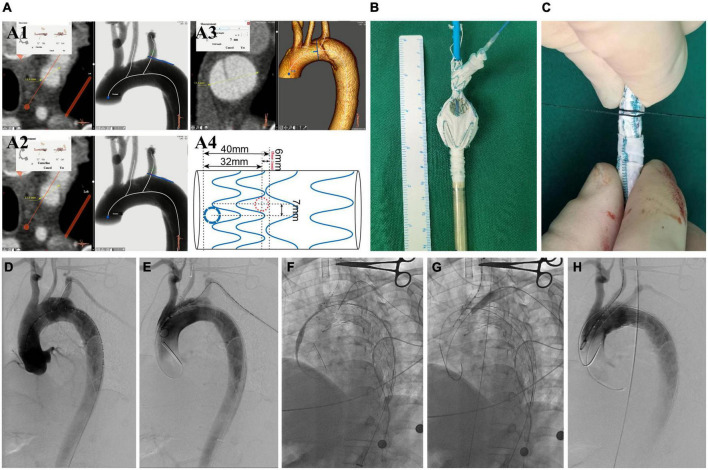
The surgeon’s details modified fenestrated stent graft and the intraoperative images of performing this endovascular technique. (A1) Measuring the diameter of LCCA and the offset angle between LCCA and LSA. (A2) The diameter of LSA. (A3) The distance between the tear entry and the proximal side of the stent. (A4) A design for preparing the modified fenestrated stent graft. (B) The unsheathed custom-made fenestrated single-branched Castor stent graft and the distal circular fenestration site are selected for LSA. (C) Photography of stent-graft when re-sheathed. (D) DSA images in the left anterior oblique view. (E) Delivery and advancement of the stent graft into the aortic arch. (F) The hydrophilic guidewire is passed cephalad into the sheath through a needle hole and advanced through the stent-graft lumen, exiting through LSA fenestration and continuing cephalad external to the stent-graft. (G) On-table covered stent was implanted and balloon dilatation. (H) DSA shows the patency of aortic arch branches and no endoleak. LCCA, left common carotid artery; LSA, left subclavian artery; CTA, computed tomography angiography; DSA, digital subtraction angiography.
